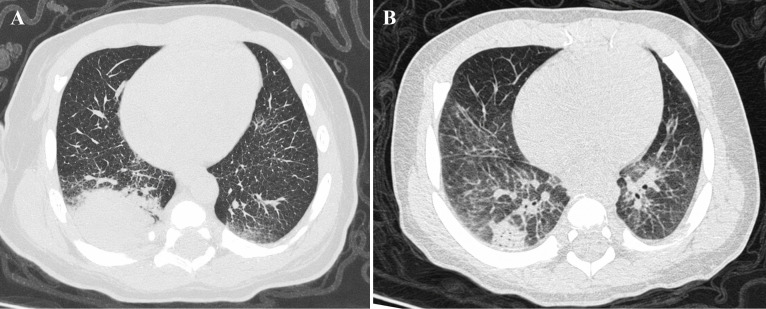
Chest computed tomography of Patient 8 (11-day-old neonate) before and after targeted therapy. (A) At admission: bilateral diffuse consolidation with possible right lung abscess formation. (B) After 14 days of azithromycin therapy combined with bronchoscopic lavage: marked improvement of the right lower lobe abscess-like lesion.
